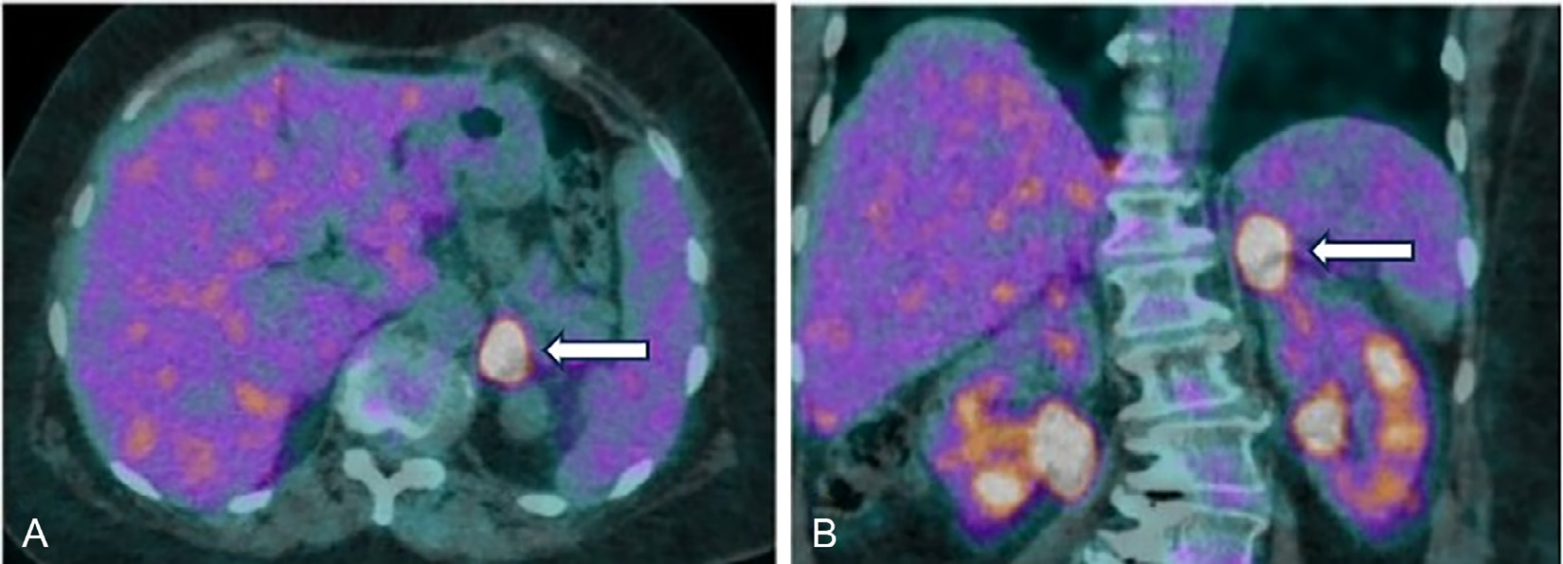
Axial and coronal fusion images from interim PET-CT after 4 cycles of CHOP and intrathecal methotrexate. Imaging reveals interval resolution of the hypermetabolic right adrenal mass and significant reduction in size and metabolic activity of the left adrenal mass, with a small residual lesion over the medial and lateral limbs.
